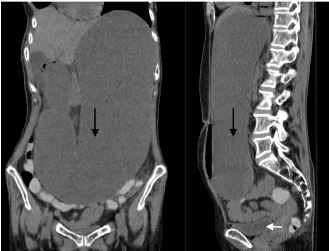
(a) Coronal computed tomography(CT) image demonstrating a markedly distended loop of bowel; (b) Sagittal CT image demonstrating a markedly distended loop of bowel, as well as partial view of the bladder. Black arrows represent the loop of bowel. White arrow points to the bladder.
